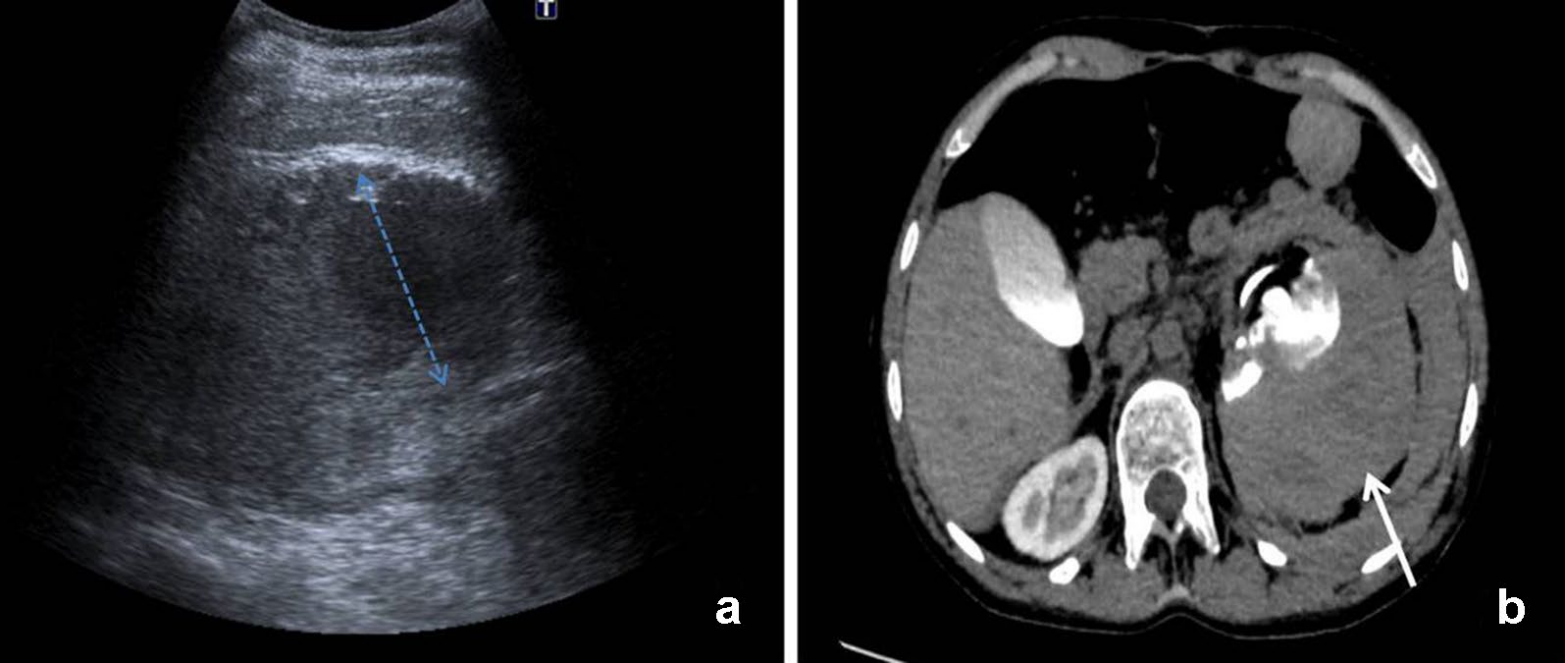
a r-EFAST: left renal hematoma of 6 cms (*arrow*). b CT Scan with intravenous contrast: perirenal hematoma (*arrow*), open to pararenal space. Also note the inhomogeneous distribution of intravenous contrast in the left kidney
